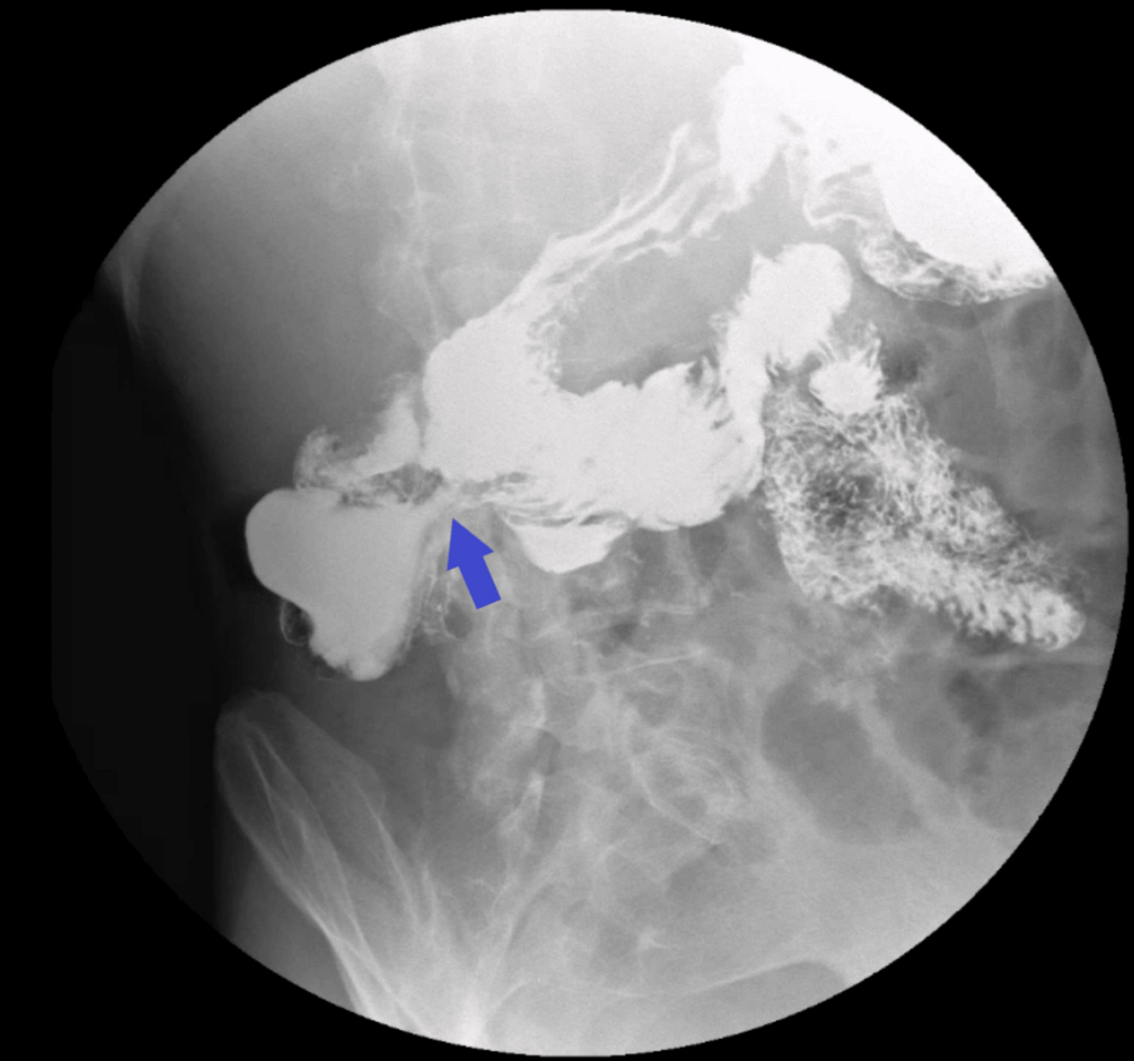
Early Contrast Passage From the Duodenum to the Colon Water-soluble contrast radiograph showing early and abnormal passage of contrast from the second portion of the duodenum to the hepatic flexure of the colon (blue arrow), confirming the presence of a duodenocolic fistula.
